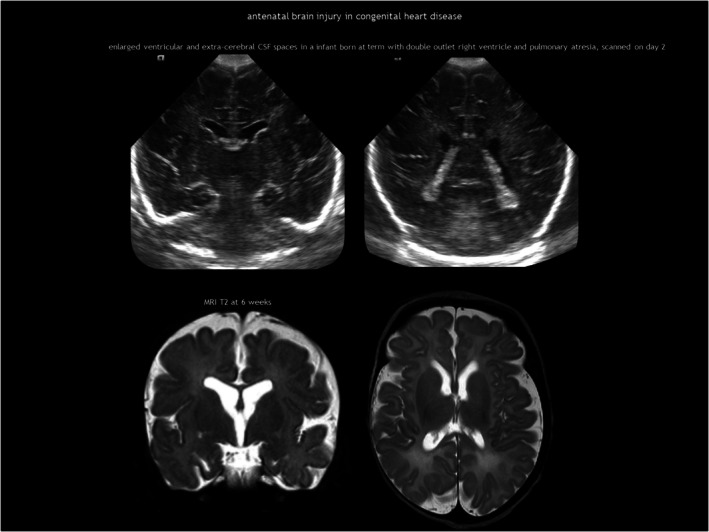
Antenatal brain injury in congenital heart disease. Abbreviations: CSF, cerebrospinal fluid; MRI, magnetic resonance imaging.
